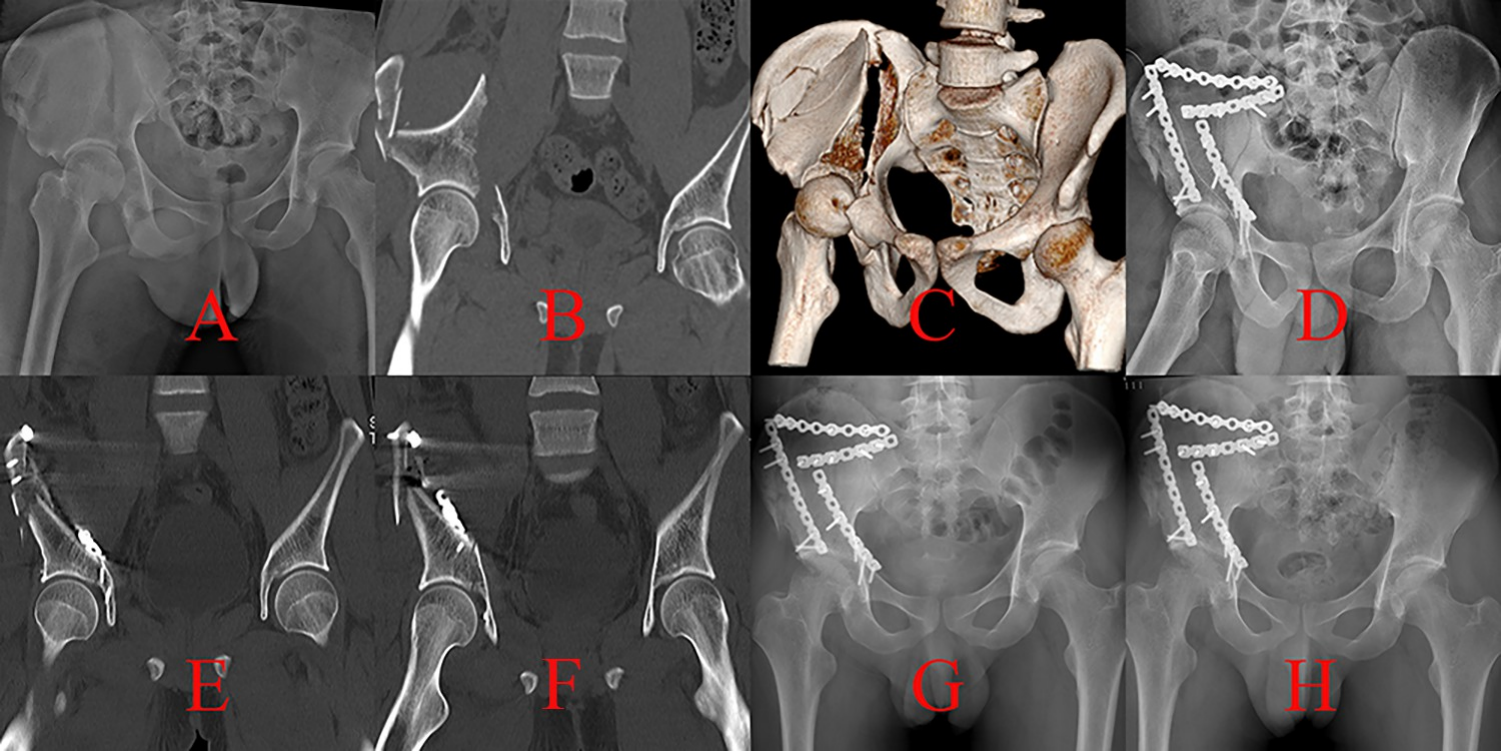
A patient with an A3.2 injury fixed with multi-plating through the iliac fossa technique was presented. A, cephalad detachment of roof column and intact iliopectineal line on preoperative AP view; B, mismatch of acetabulum (a larger diameter) and femoral head; C, cephalad and separated detachment of roof column on 3D reconstruction; D, excellent reduction quality on postoperative projection; E-F, postoperative CT scans showed excellent concentric reduction of acetabulum and femoral head; G, AP view a month postoperatively; H, fracture lines almost disappeared on AP view 3 months postoperatively.
